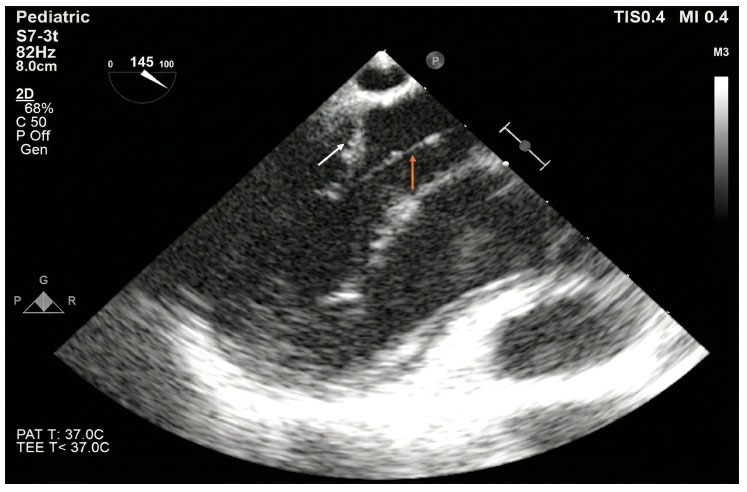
A transesophageal echo at 145 degrees showing the false tendon (orange arrow) during diastole extending from the left atrium to the left ventricle. Mitral valve (white arrow).
